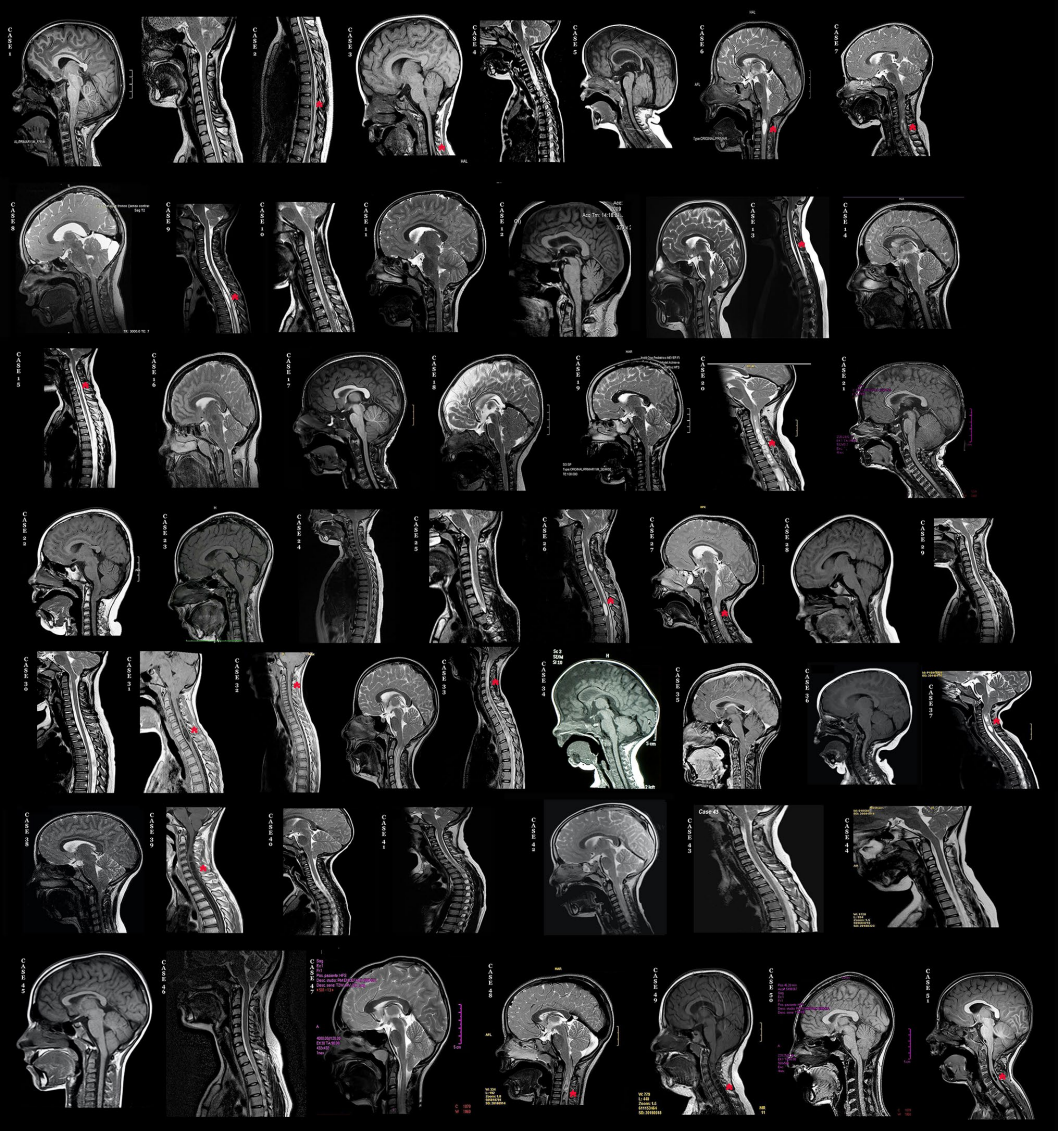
Sagittal T1 or T2-weighted MRI images showing Chiari type I malformation (C1M) in our 51 cases as evidenced by low-lying cerebellar tonsils. Sagittal MRI of the cervical spine also detects different cases of syringomyelia indicated by red asterisks
